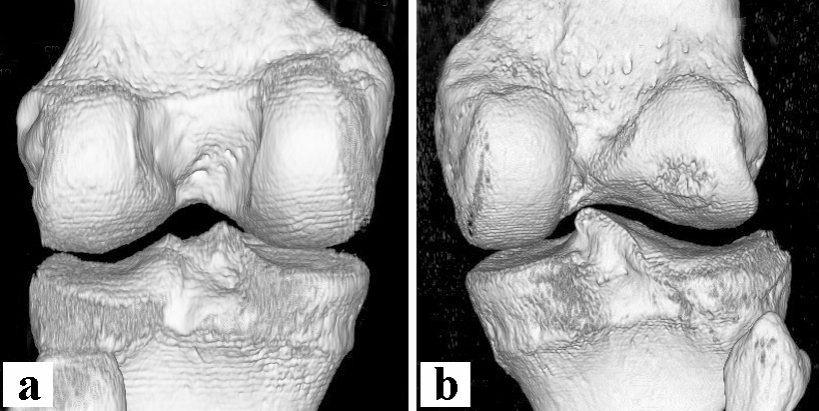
Three-dimensional reconstruction of CT scans. Posterior view on three-dimensional reconstruction of CT scans of the contralateral (a) and the affected (b) knee. b shows the extremely narrow notch and the deformity of the lateral femoral condyle. Note the malformation of the tibial eminence with only one spine (b) as opposed to the normal tibial eminence with two spines (a).
